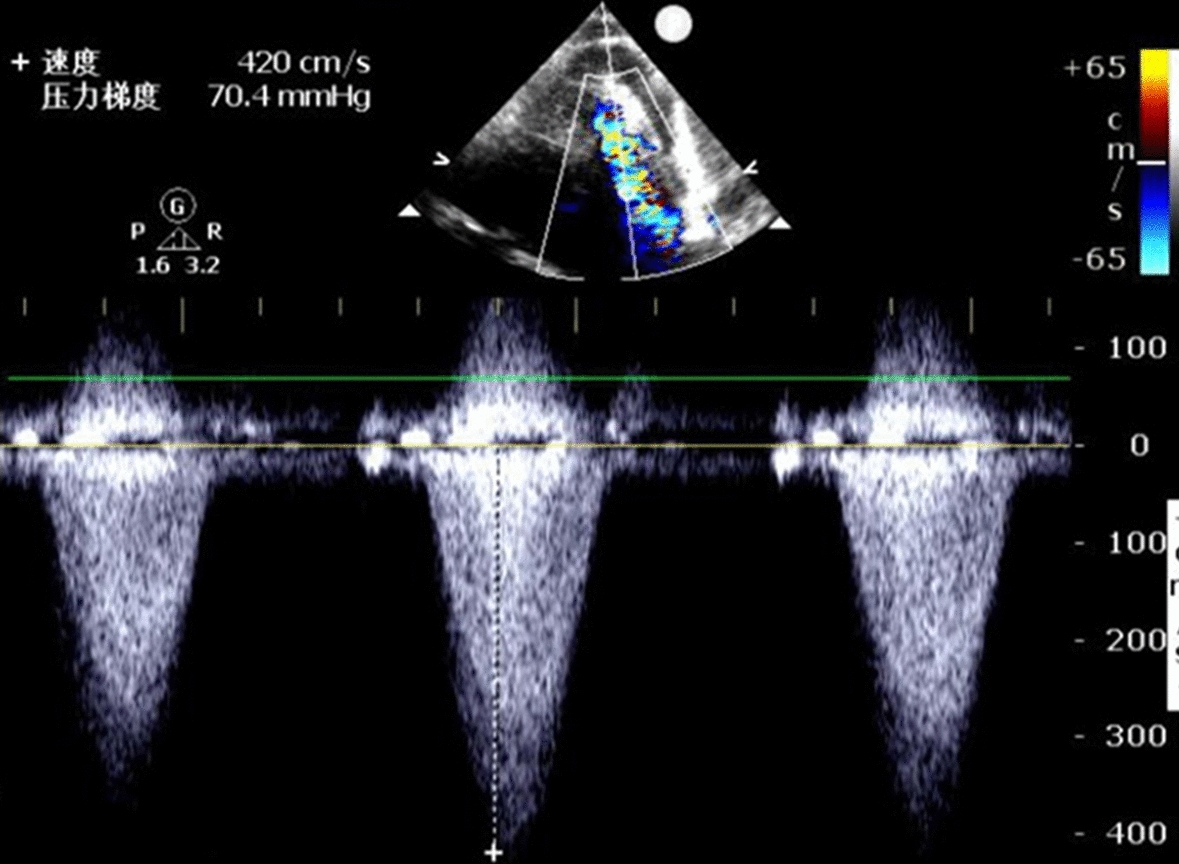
Severe tricuspid regurgitation on spectral Doppler
